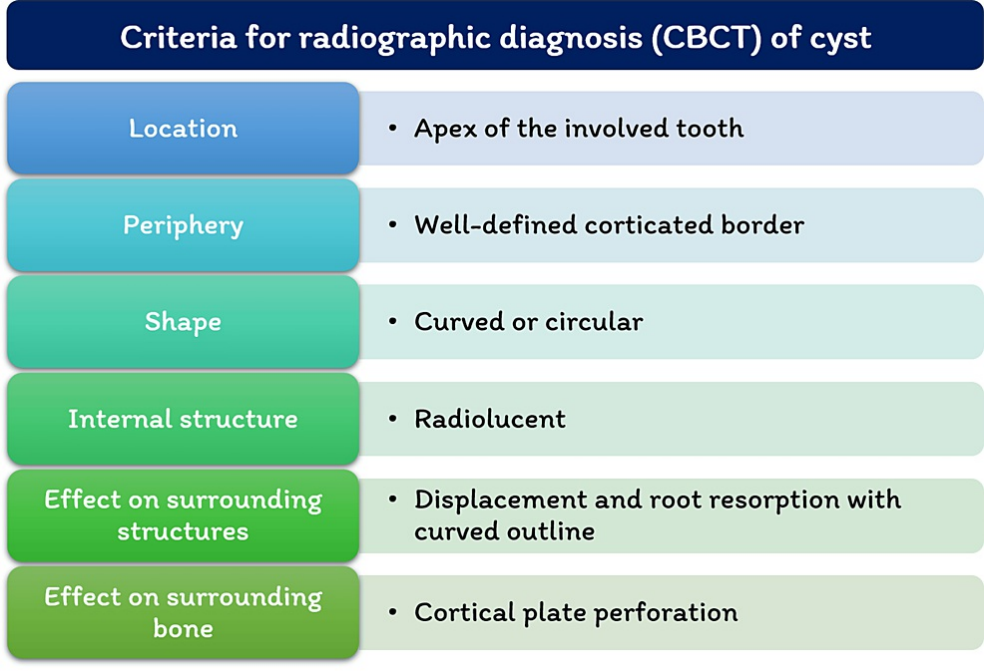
Criteria for radiographic diagnosis of radicular cyst CBCT- Cone-beam computed tomography Image Credits: Paridhi Agrawal
